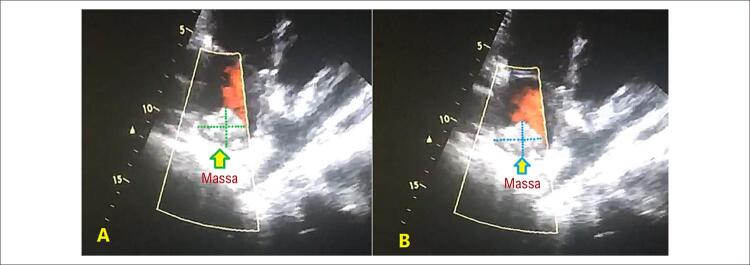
ETT por Doppler colorido, evidenciando uma massa extracardíaca invadindo o AD e exercendo efeitos compressivos sobre a parte distal da VCS. ETT: ecocardiograma transtorácico; AD: átrio direito; VCS: veia cava superior.
